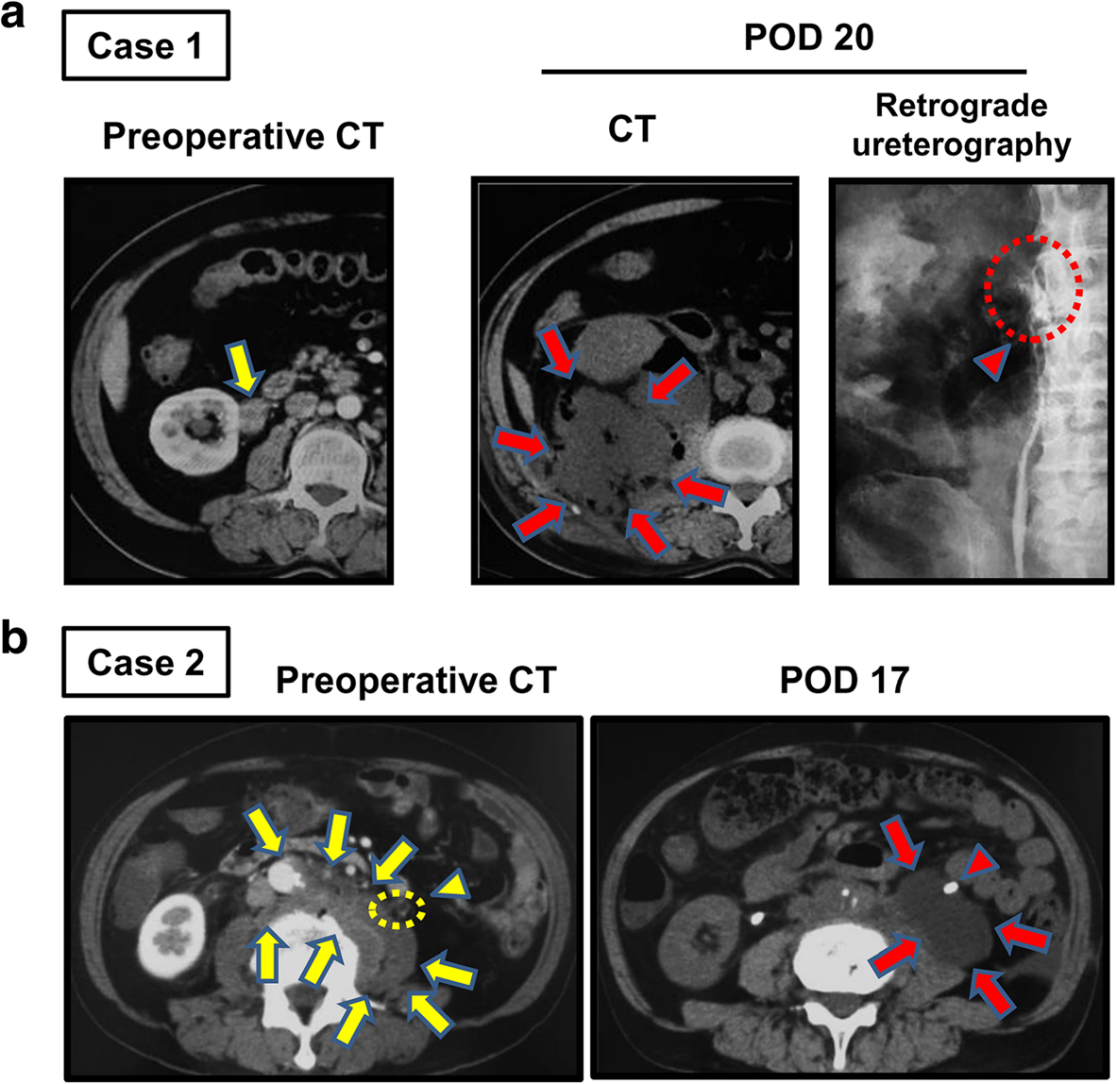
Two cases experiencing retroperitoneal urine leakage after retroperitoneoscopic tissue biopsy. a Case 1. Contrast enhanced CT scan of a 67-year-old man demonstrates extra-urinary lesions of the right ureter (yellow arrow), which results in ureteral obstruction and hydronephrosis. The CT scan on postoperative day (POD) 20 shows an infectious fluid storage in the retroperitoneal cavity (red arrows). Ureteral injury at the biopsy site is detected by the transurethral retrograde ureterography (red arrowhead). b Case 2. Contrast-enhanced CT scan of a 64-year-old man demonstrates retroperitoneal lesions involving aorta, inferior vena cava, common ileac arteries, and left psoas (yellow arrows). The left ureter is located close to the lesion (yellow dashed line and arrowhead). The CT scan on POD 17 shows a cystic urinoma in the retroperitoneal cavity (red arrows). The red arrowhead indicates the ureter with a ureteral stent
